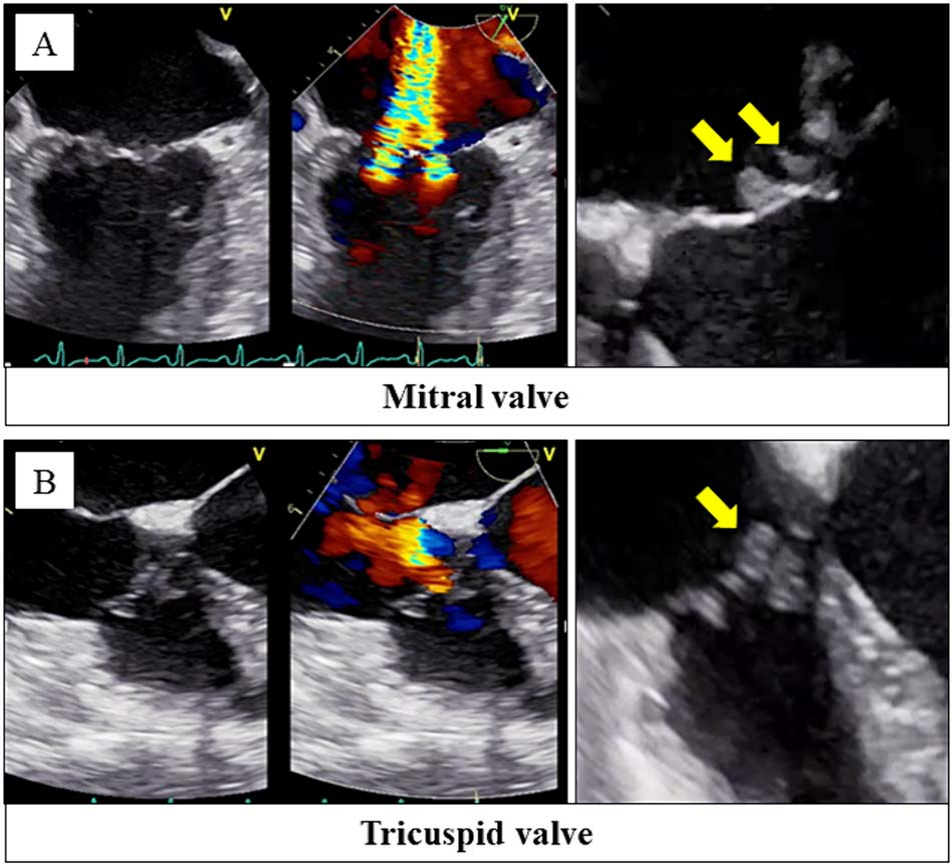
Transesophageal echocardiography revealed severe mitral regurgitation and tricuspid regurgitation with mobile vegetations on both valves (A, B).
